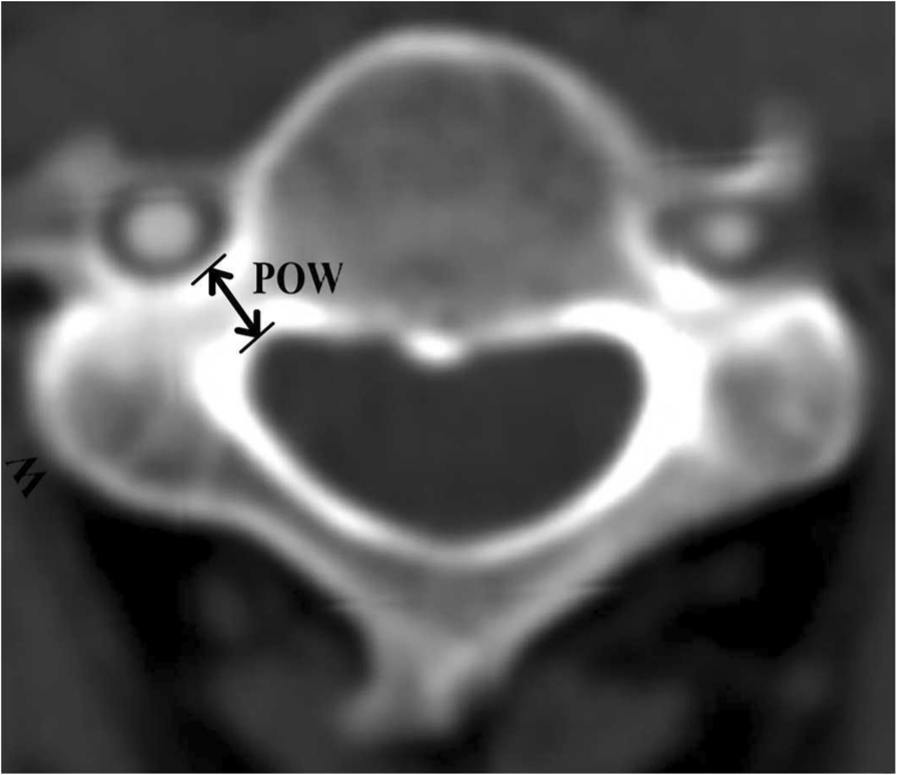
Measurement of pedicle outer width (POW) on transverse reconstruction images of computed tomography. POW indicated the mediolateral diameter of the pedicle isthmus, perpendicular to the pedicle axis
